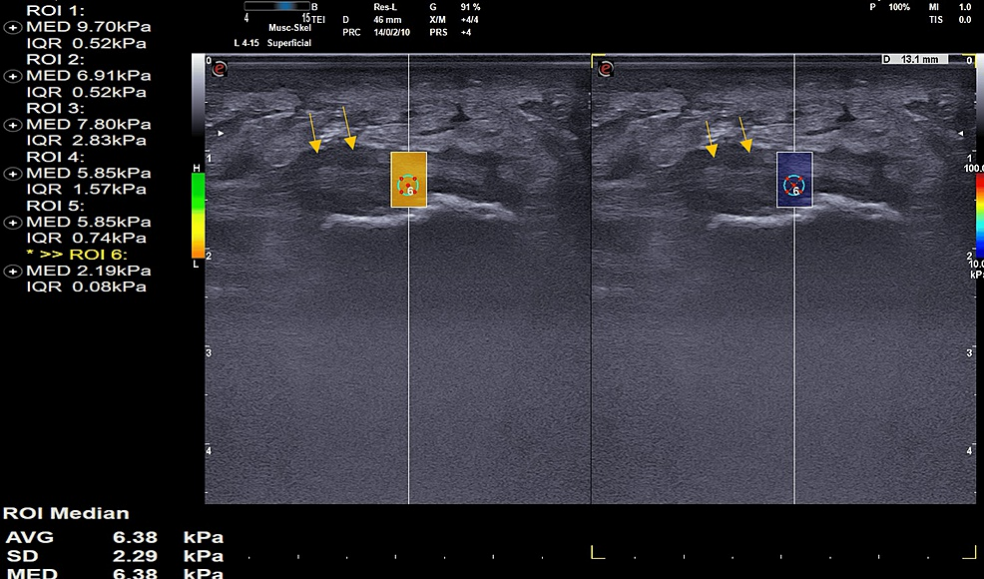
Plantar fasciitis: Sagittal B-mode ultrasound shows thickened, hypoechoic plantar fascia with loss of the fibrillar pattern (arrow showing the region of interest). The longitudinal SWE ultrasound image of a 38-year-old patient with plantar fasciitis demonstrates a stiffness of 6.38 Kpa. Kpa: Kilo Pascals
